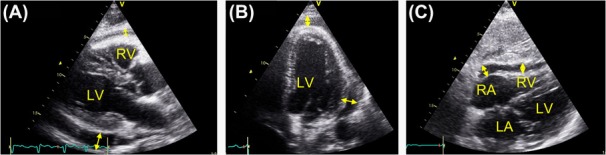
Echocardiography with pericardial effusion. A: parasternal long axis view. B: apical four-chamber view. C: subcostal view. *Double arrows* indicate the measure points of pericardial effusion. LV, left ventricle; RV, right ventricle; LA, left atrium; RA, right atrium.
